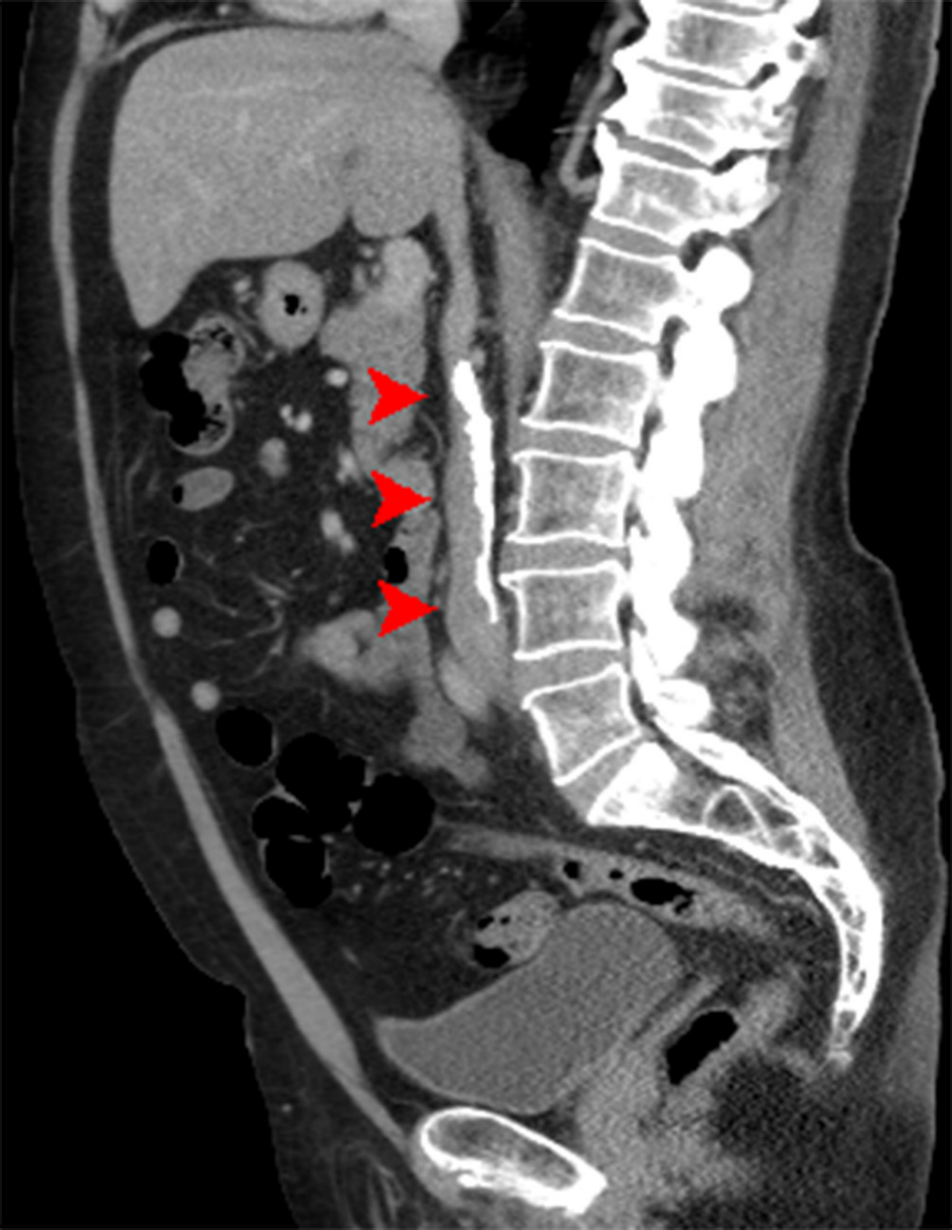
Sagittal CT showing calcification of the IVC. IVC, inferi orvena cava.
